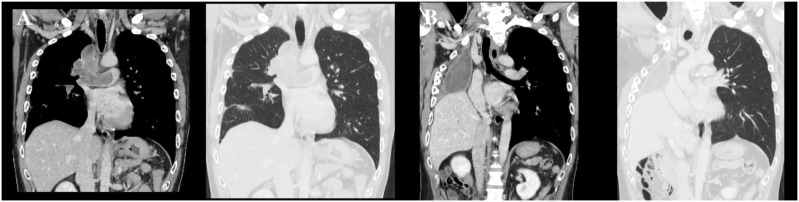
(A) Preoperative contrast-enhanced CT in the coronal plane showing an intravascular tumor extending within the pulmonary artery. (B) Postoperative contrast-enhanced CT in the same coronal plane demonstrating disappearance of the intravascular lesion after surgical resection.
